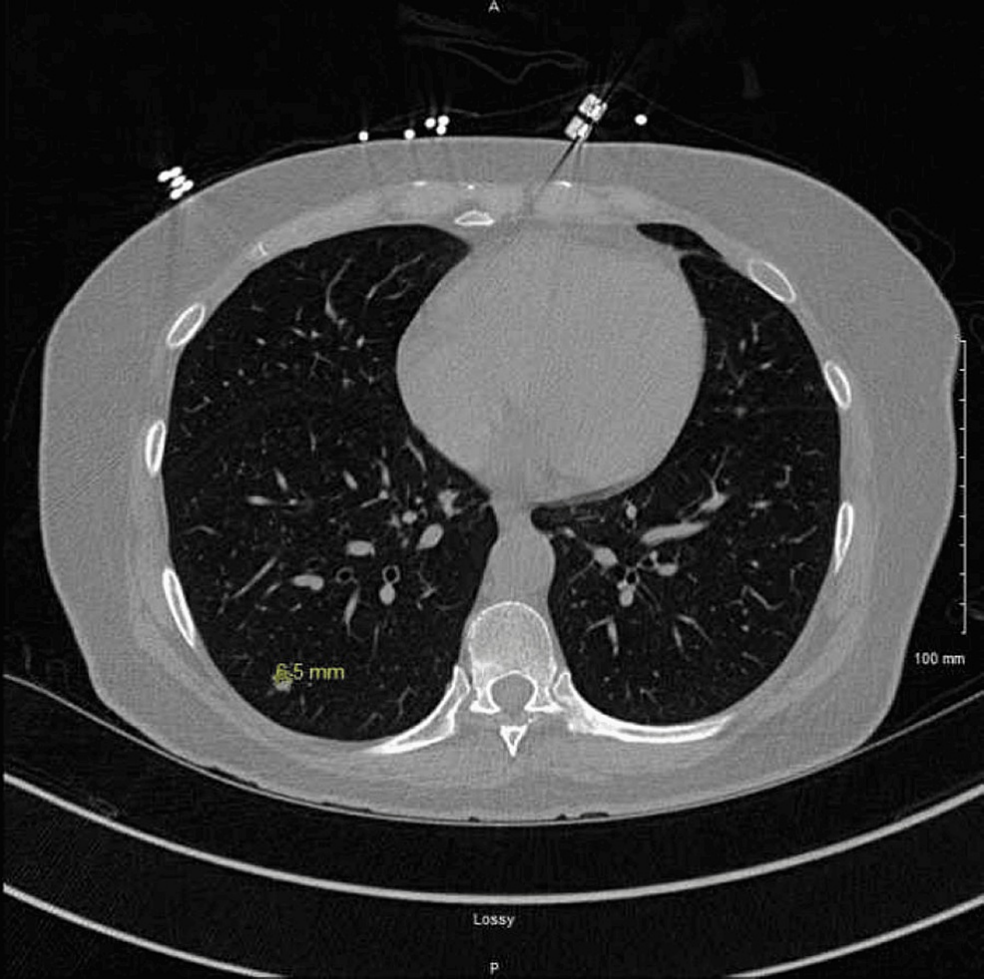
CT chest showing 6.5 mm pulmonary nodule. Multiple nodules were unmarked.
